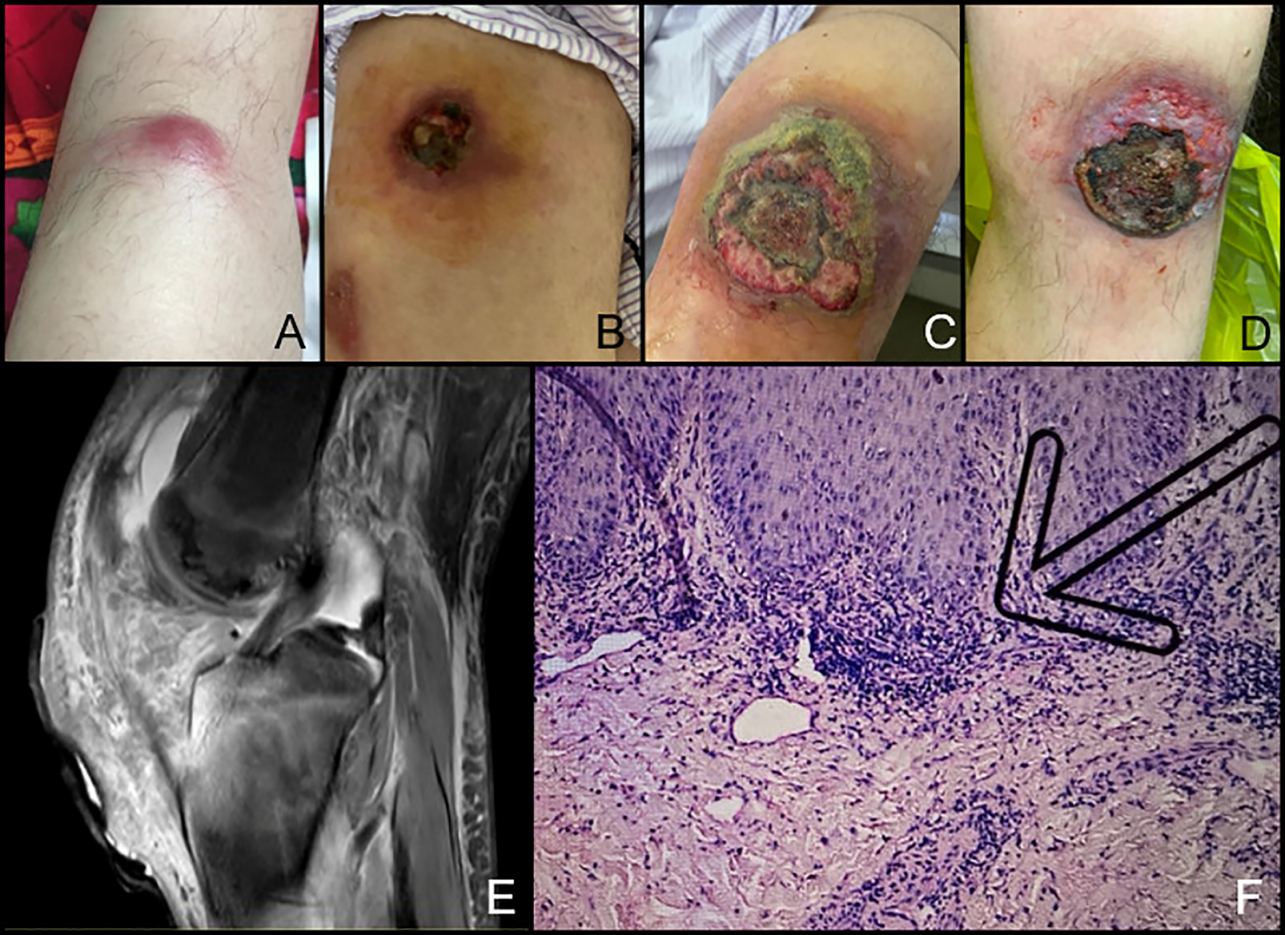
Skin lesion and arthritis of the Chinese patient with APLAID. (A) The lump under the left knee; (B, C). The ulceration which was diagnosed as gangrenous pyoderma got worse. (D) Ulceration healing after dressing changing and adalimumab treatment. (E) Bone marrow erosion of the knee on MRI. (F) Skin biopsy of the ulceration. The arrow indicates perivascular lymphocyte infiltration.
